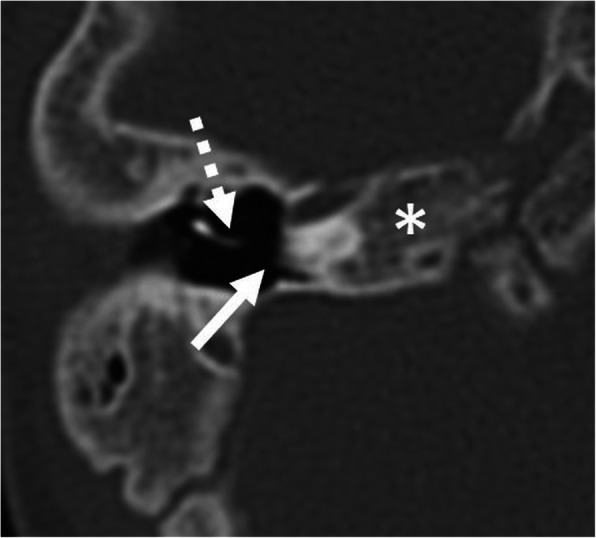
A 1-year-old male patient, with bilateral sensorineural deafness from birth. HRCT axial image shows hypoplastic right petrous bone with a complete absence of the inner ear structures (asterisk), compatible with Michel’s deformity. The medial wall of the middle ear is flat (arrow). Absent round and oval windows. Absent stapes. Normal-looking malleus (dashed arrow)
